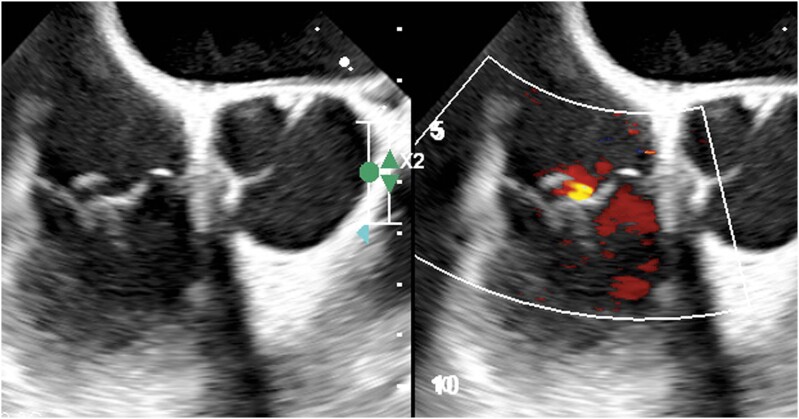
Two-dimensional (2D) trans-oesophageal echocardiogram, right ventricular outflow tract view. Tricuspid valve leaflets appear redundant and prolapsing with associated mild regurgitation.
